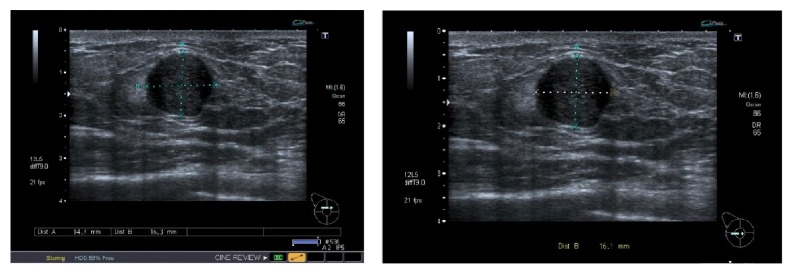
Ultrasound scan showing the lesion 15 × 16 mm mass (U3).
